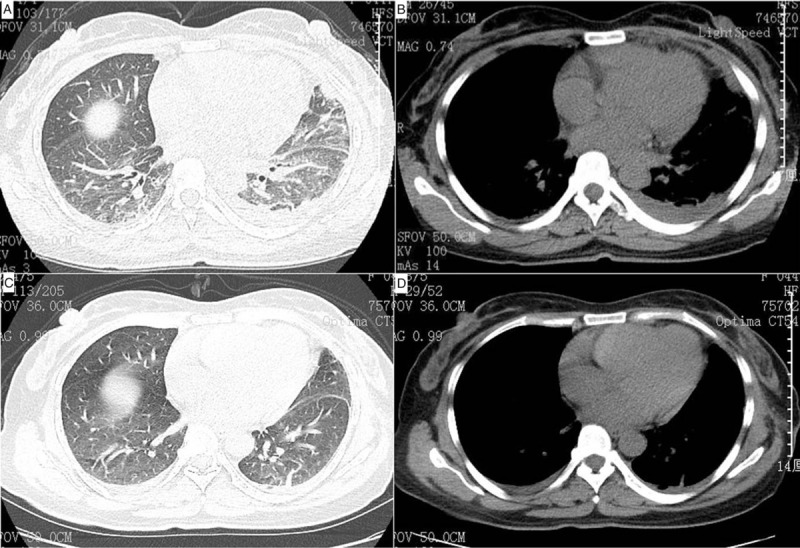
(A and B) Chest computed tomography (CT) showed pulmonary infection, bilateral pleural effusions, and left pleural hypertrophy. (C and D) CT showed the pleural effusion was almost absorbed after 2 courses of azacitidine treatment.
